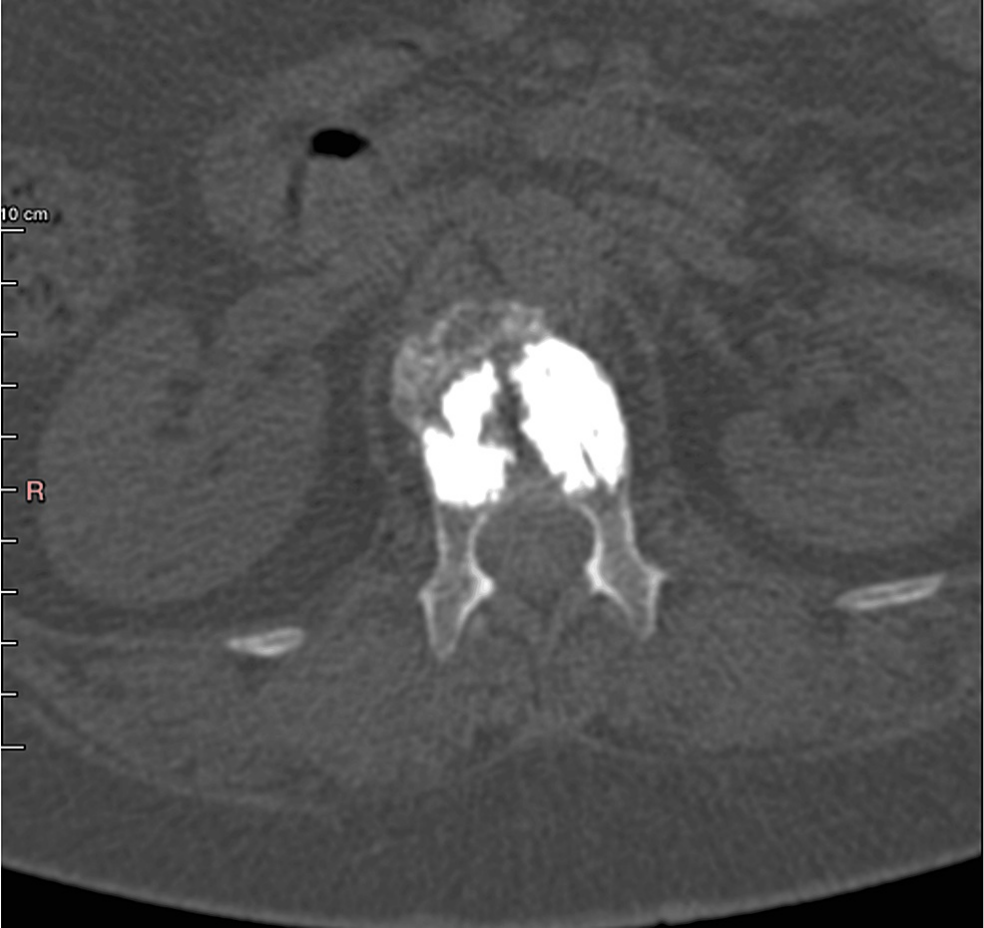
CT-scan (axial view) of the lumbar spine obtained immediately following the two balloons kyphoplasty performed on the L1. No gross signs of a malignant tumor and/or breaching of the vertebral cortex are apparent.
